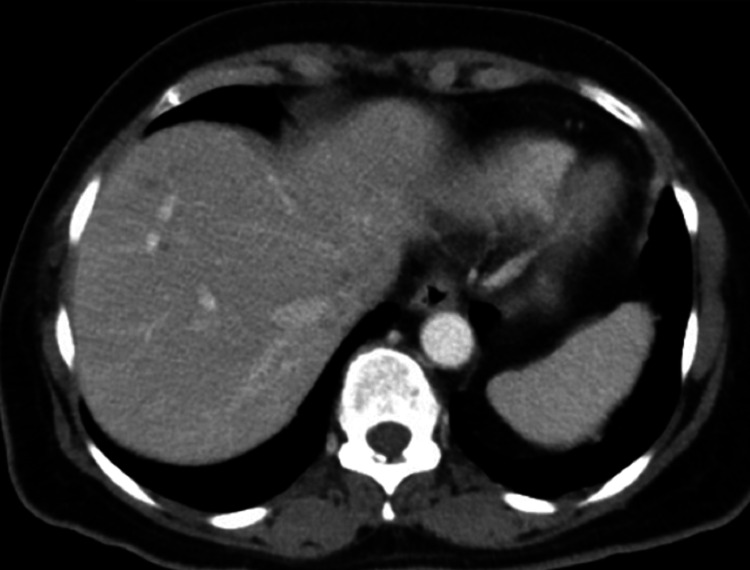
Repeat CT A/P showing near complete resolution of all hepatic lesions at approximately six weeks CT A/P: computed tomography of the abdomen and pelvis
